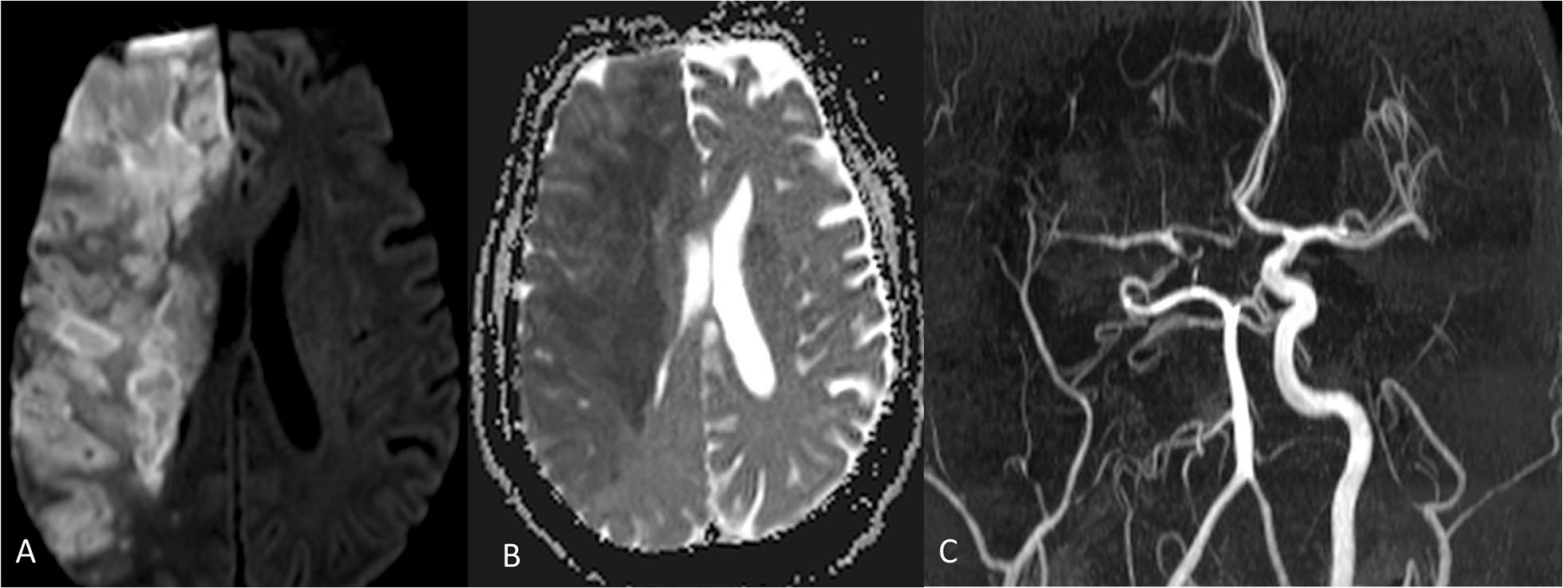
A (DWI) and B (ADC) images show acute infarct in right internal artery territory. C (MR angiography thick slab image): There is non-visualization of right internal carotid artery. Carotid Doppler (not shown) revealed a thrombus in proximal right internal carotid artery. Right middle cerebral artery is seen to be partly reformed by collaterals
